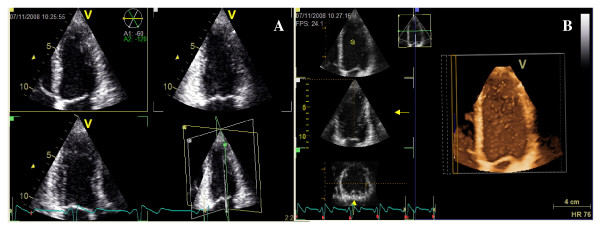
Triplane echocardiography: 4 – 2 -and 3 chamber views are simultaneously displayed with 4-chamber as the reference view. Interplane angles are set at 60 degrees (A). A full volume acquisition of left ventricular during four cardiac cycles (B).
